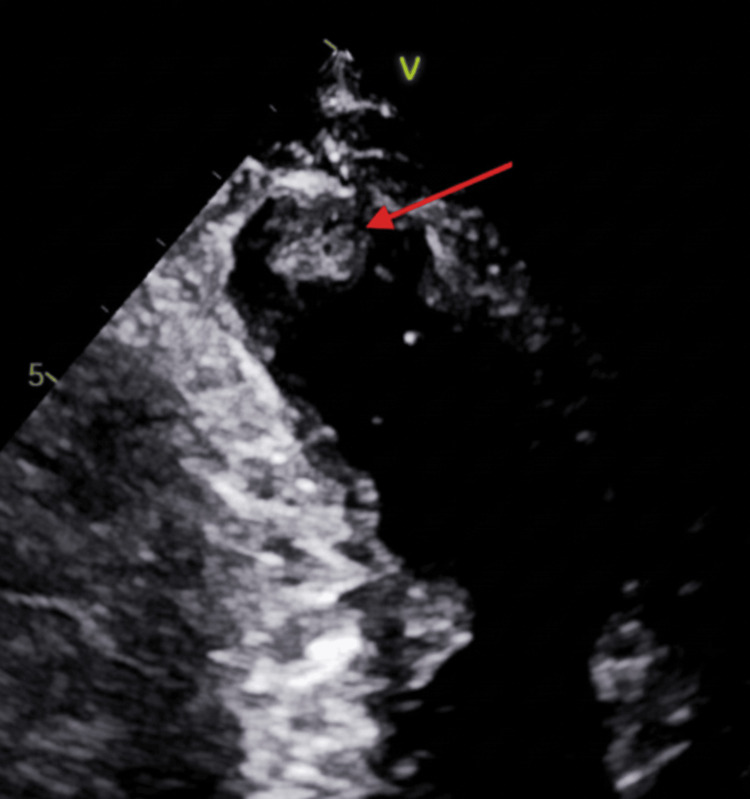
Follow-Up Echocardiogram Demonstrating Apical Aneurysm and Thrombus Formation Follow-up transthoracic echocardiogram demonstrating aneurysmal remodeling of the left ventricular apex, with the red arrow indicating a small apical thrombus.
